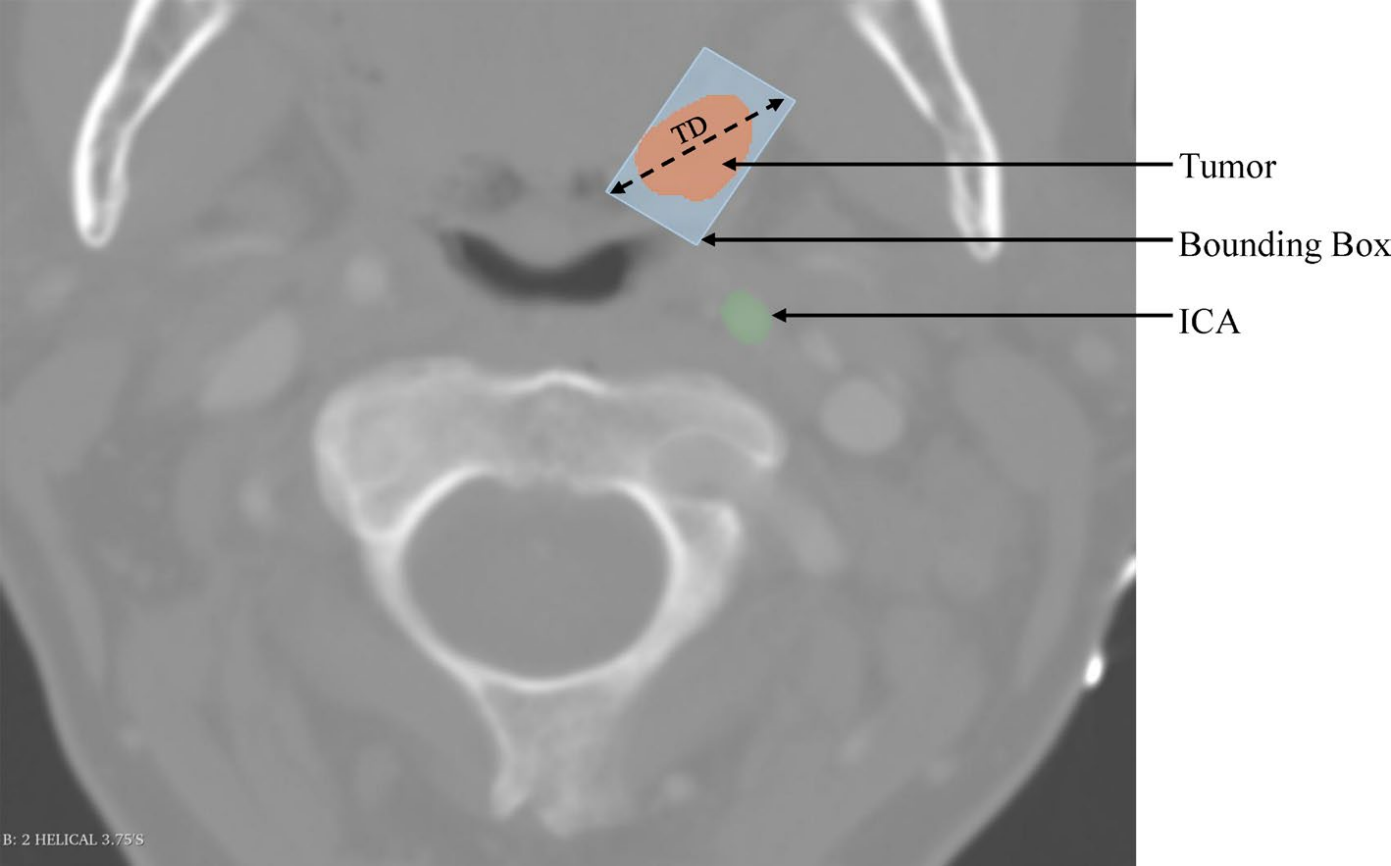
CT scan of tonsillar tumor (orange) and internal carotid artery (ICA, green). Bounding box (light blue) around tumor with transverse plane diameter (TD, dashed arrow) are depicted. [Color figure can be viewed at wileyonlinelibrary.com]
